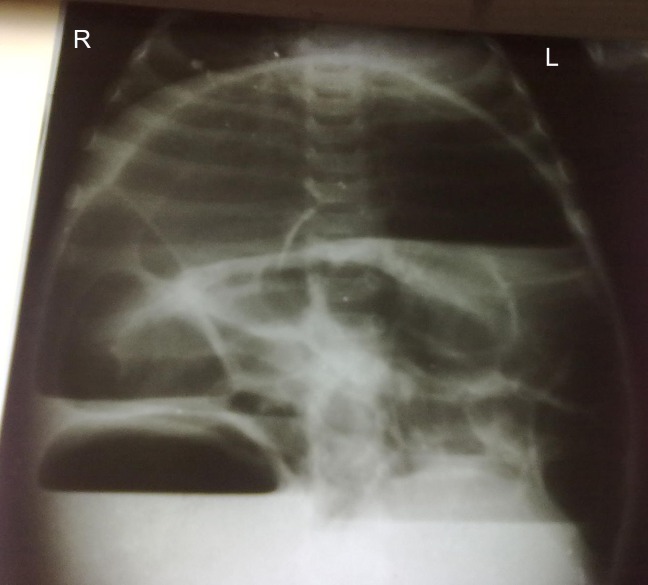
Figure 1: Xray abdomen erect posture showing air fluid levels indicating intestinal obstruction.
